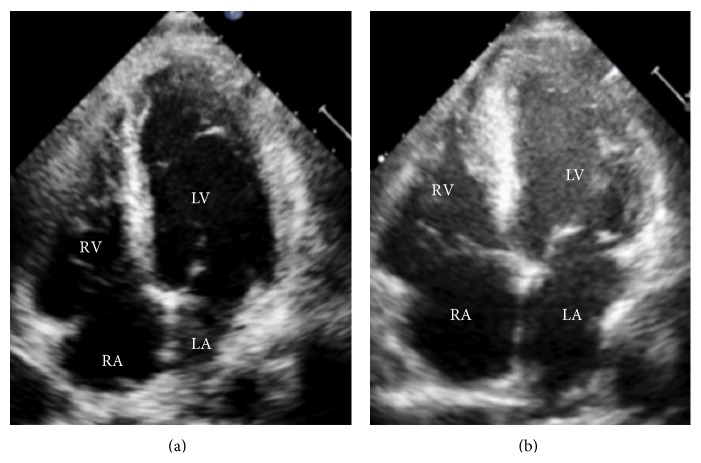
Transthoracic echocardiogram (apical four-chamber view). (a) Hospital day 1: normal ventricular function, normal wall thickness. (b) Hospital day 7: left ventricular systolic function is severely reduced, wall thickness is increased, and echocontrast is present in the left ventricle consistent with stasis of blood flow. LV: left ventricle, RV: right ventricle, LA: left atrium, and RA: right atrium.
